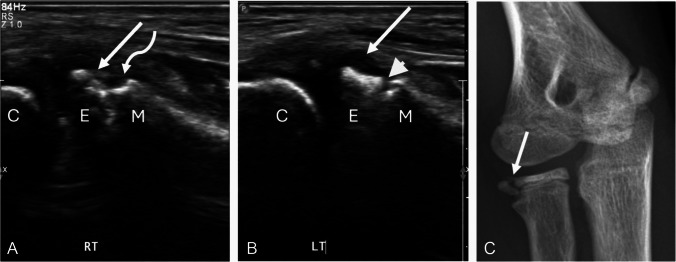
An 11-year-old football player presented 3 months after a helmet impact to the right elbow sustained during a game. The initial clinical concern was a ligamentous injury, prompting an ultrasound evaluation. Sonography of the lateral elbow demonstrates irregularity of the radial head articular cartilage and subchondral bone (*arrow*) as well as adjacent metaphyseal irregularity (*curved arrow*). Normal anatomy is seen on the contralateral left (*LT*) side with bony landmarks: capitellum (*C*), radial epiphysis (*E*), and radial metaphysis (*M*). The articular cartilage (*arrow*) and physis (*arrowhead*) appear normal. Subsequent radiographs of the right elbow demonstrate a radial head fracture extending to the physis
